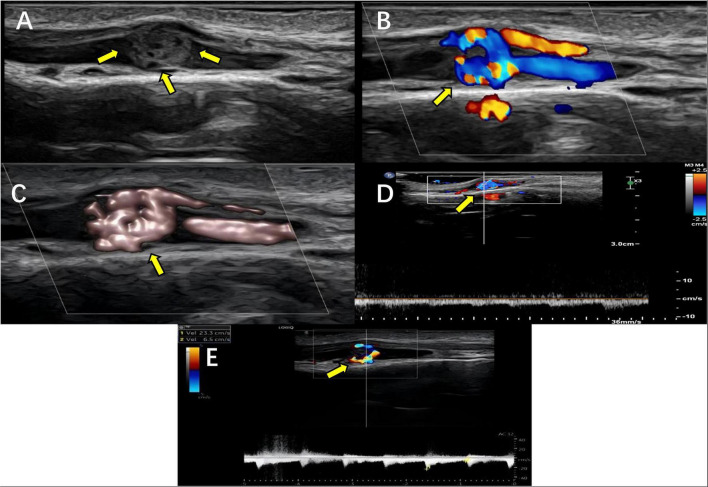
Multimodal ultrasonic manifestations of benign glomus tumor in subcutaneous superficial vein. (A) Grayscale ultrasound. (B) Color Doppler imaging. (C) Power Doppler imaging. (D) Venous spectrum was detected within the mass. (E) Arterial spectrum was detected in the mass.
